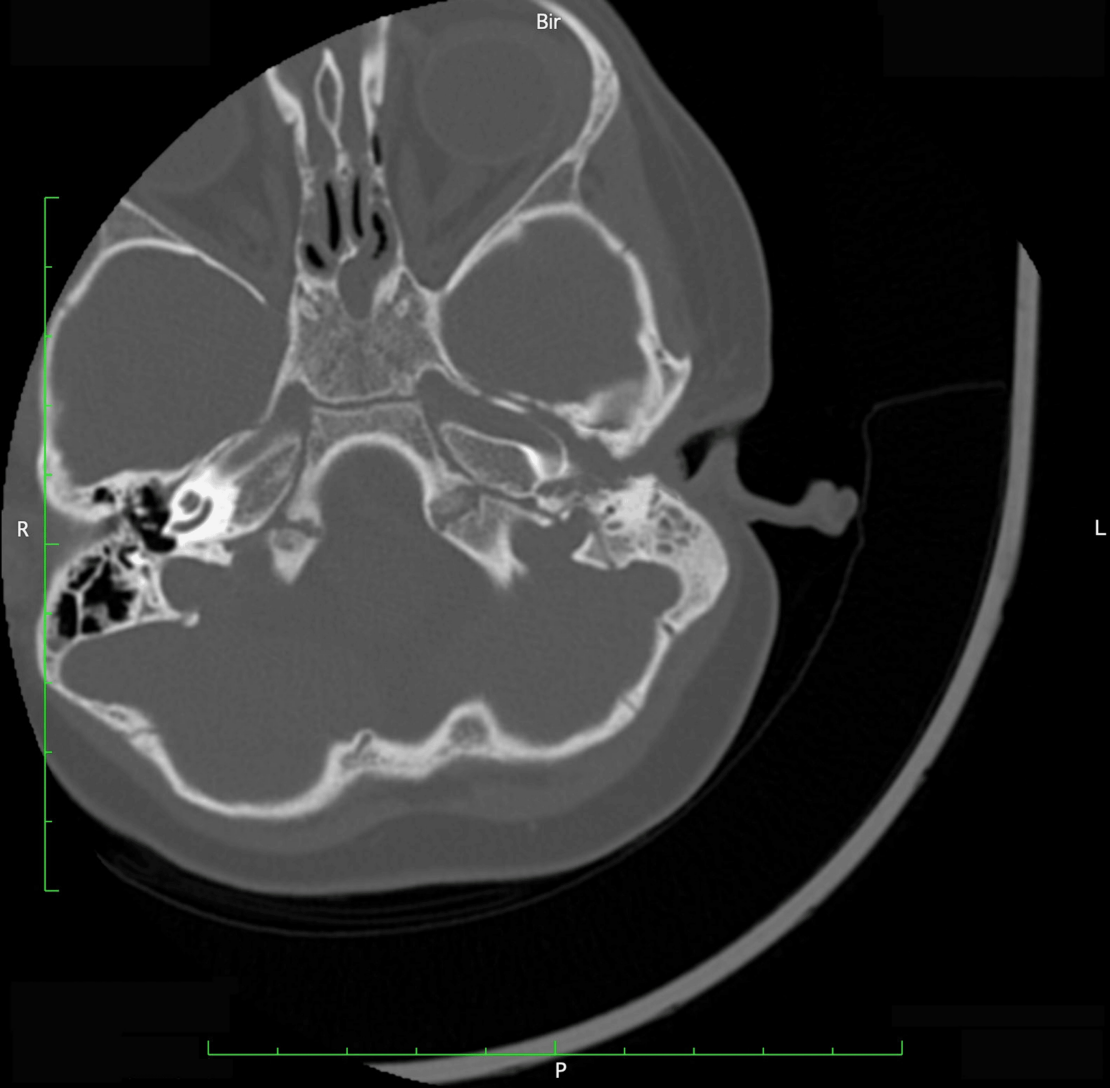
A computed tomography scan of the head Left-sided middle ear and mastoid effusion with complete opacification of the mastoid air cells. Notably, the tympanic cavity showed no erosion of the bony walls in the middle ear or external auditory canal. (L-left; R-right)
